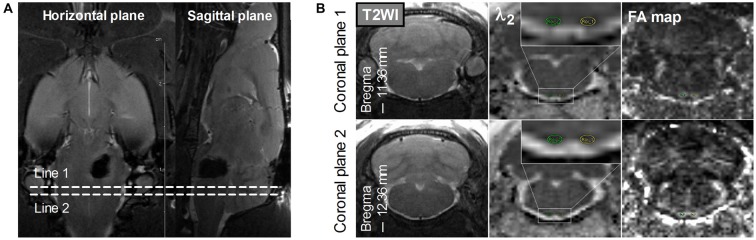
Definition of the regions of interest (ROIs) of the bilateral pyramidal tracts (PYs). (A) The definition of the PY (bregma, −11.36 mm to −12.36 mm) caudal to the hematoma on the horizontal and sagittal plane. Line 1 and Line 2 perpendicular to the horizontal and sagittal planes represent two planes that are located at –11.36 mm and –12.36 mm of bregma, respectively. (B) The definition of the bilateral PYs on two coronal planes. The images in the left column are coronal T2-weighted images. The images in the middle column are coronal λ_2_-weighted images that show the definition of the ROIs of the bilateral PYs (encircled in green and yellow and enlarged in the insets). The images in the right column are the corresponding Fractional anisotropy (FA) maps.
